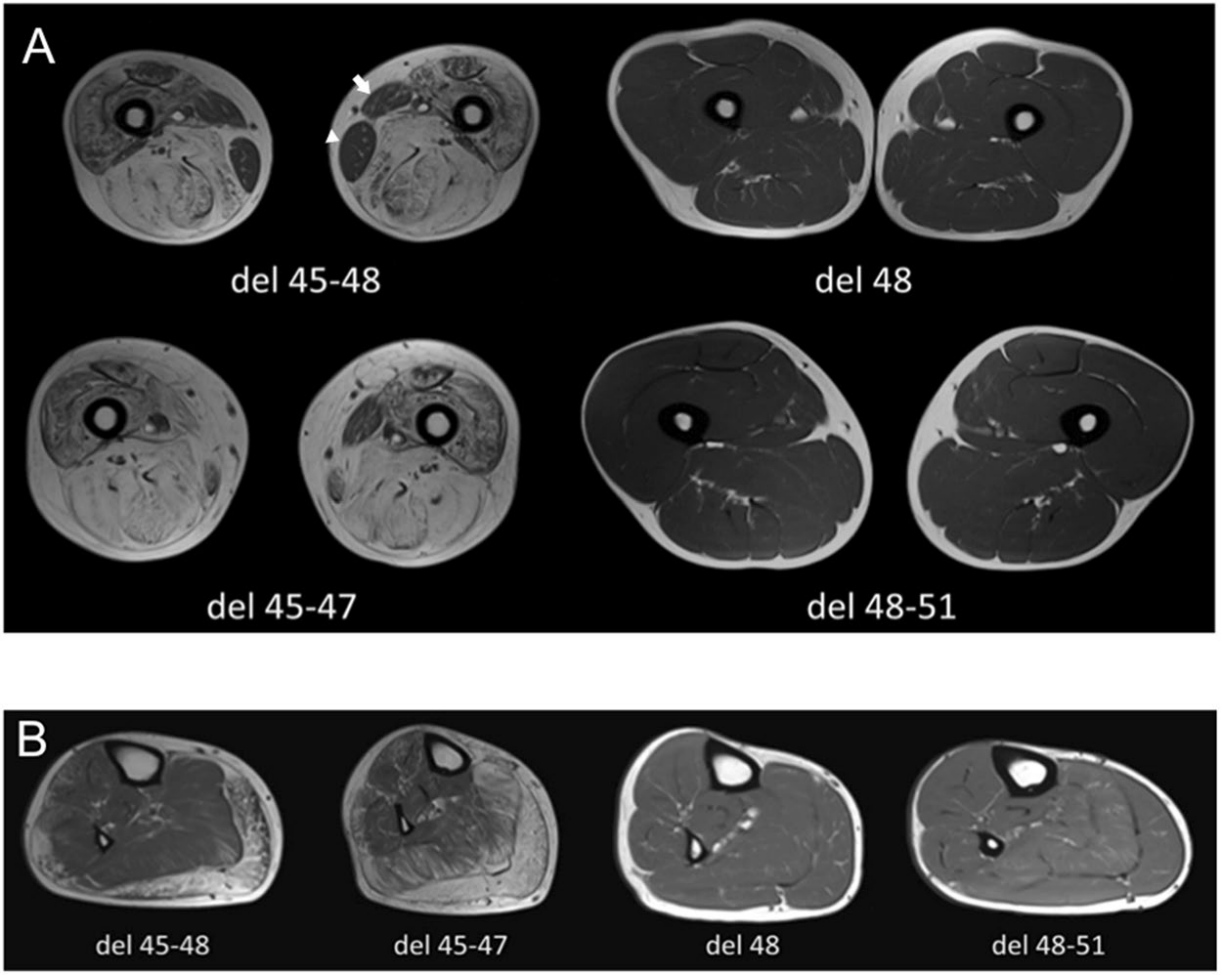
Representative T1w MRI scan images. (A) Thigh MRI scan (T1w sequence) of four BMD patients (aged 40–42 years) with different genotypes. Patients with del 48 and del 48–51 mutations showed a normal signal without signs of fatty replacement. On the contrary, patients with del 45–47 and del 45–48 mutations showed severe fatty replacement, in particular of the gluteus maximus (Mercuri score 2b-4). Note the sparing of the gracilis (white arrowhead) and sartorius (white arrow) muscles. (B) Leg MRI scan (T1w sequence) of four BMD patients (aged 40–42 years) with different genotypes. Patients with del 48 and del 48–51 mutations showed a normal signal without signs of fatty replacement, while patients with del 45–47 and del 45–48 mutations showed advanced fatty replacement, in particular of the gastrocnemii (Mercuri score 2b-3), and a moderate involvement of soleus, peroneus, and tibialis anterior (score 2b). Conversely, muscle MRI showed a lesser involvement of the tibialis posterior (Mercuri score 0–1).
